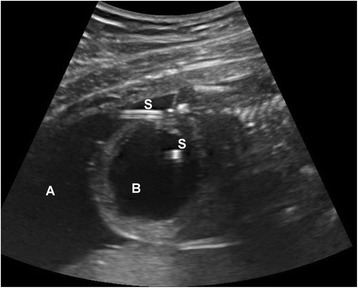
Ultrasound image of a vesicoamniotic shunt with the two catheter ends (S) in the fetal bladder (B) and amniotic cavity (A)
